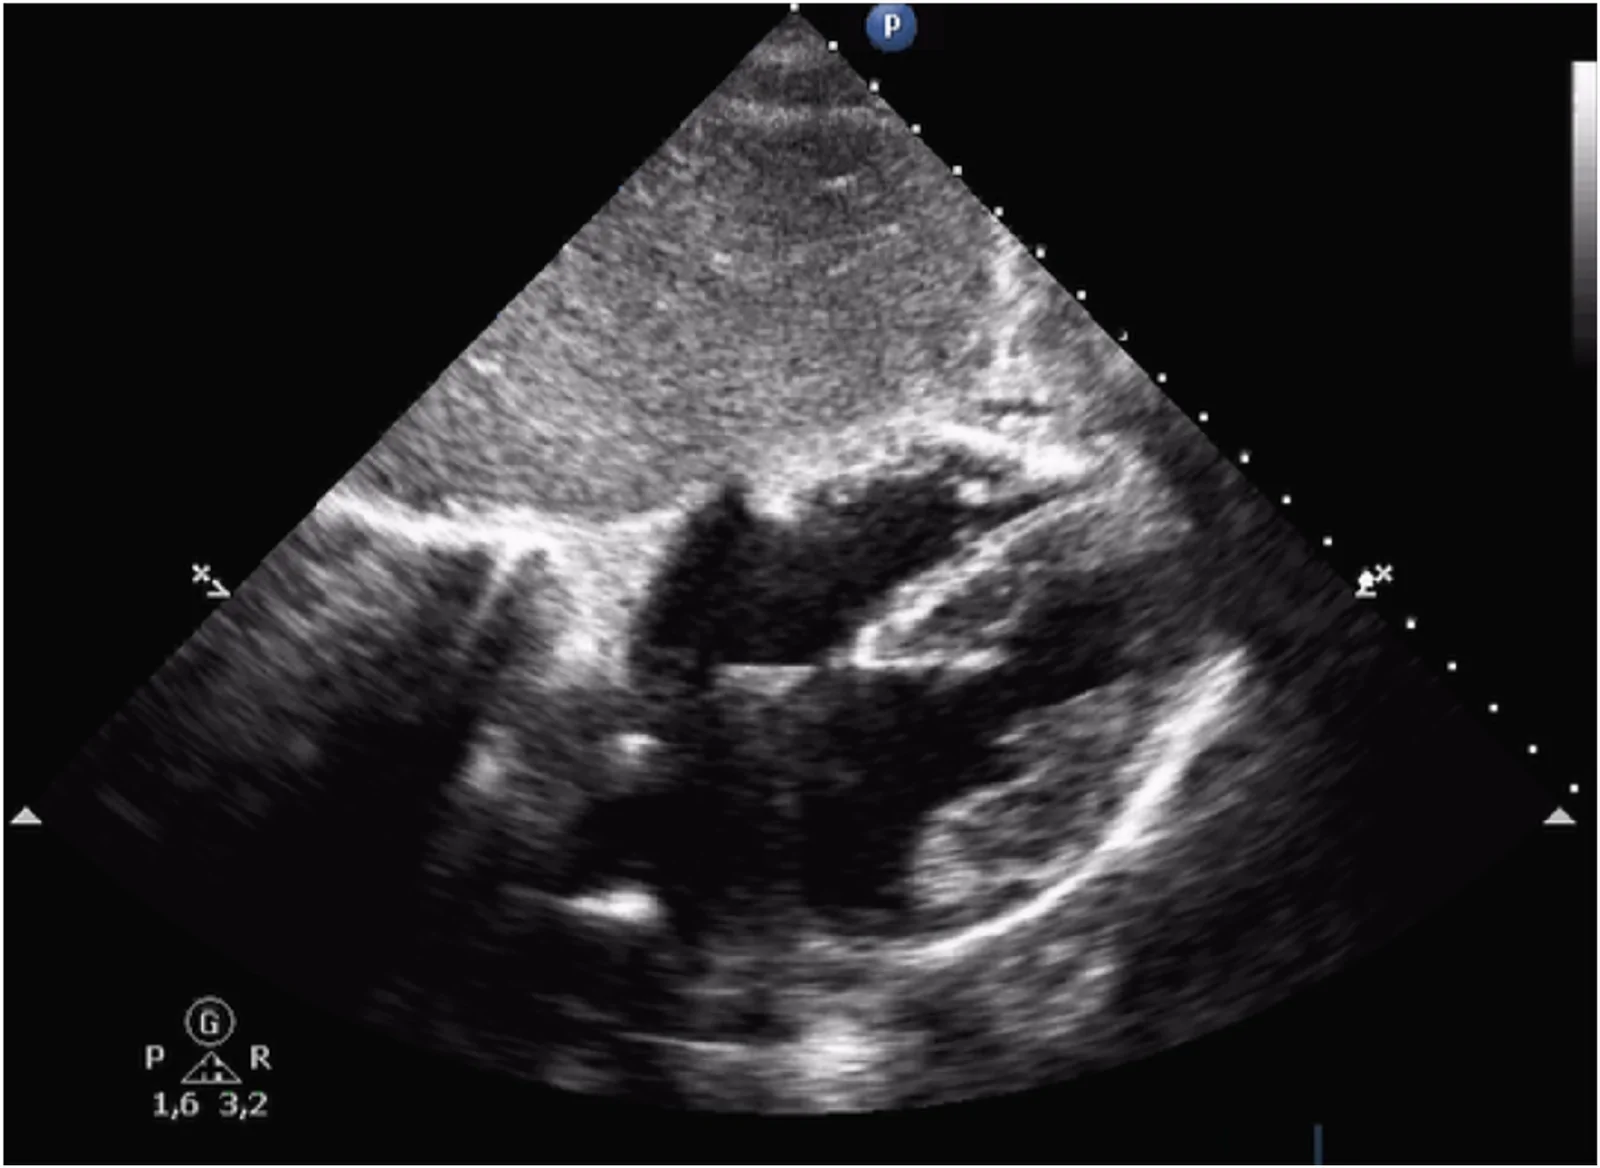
Follow-up transthoracic echocardiography obtained from a subcostal view 8 days after initiation of thyroid hormone replacement therapy, demonstrating marked reduction of the circumferential pericardial effusion from 8 mm to approximately 2 mm.
